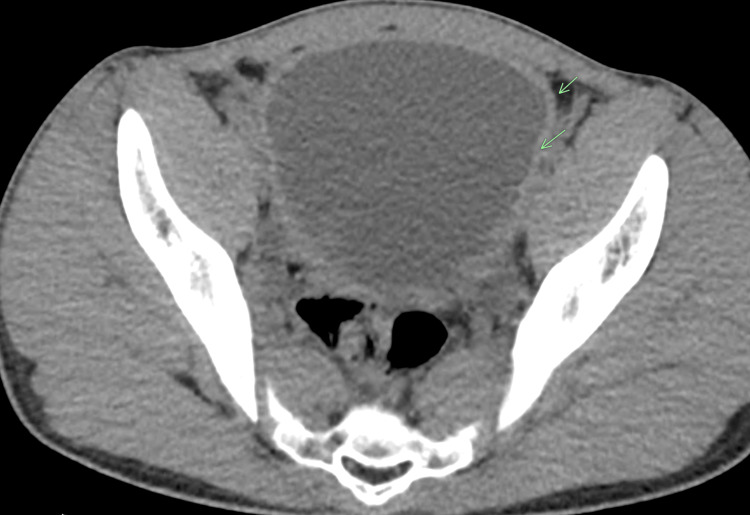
Computed tomography (CT) of the abdomen and pelvis Axial non-contrast CT image of the pelvis demonstrated urinary bladder wall thickening.
